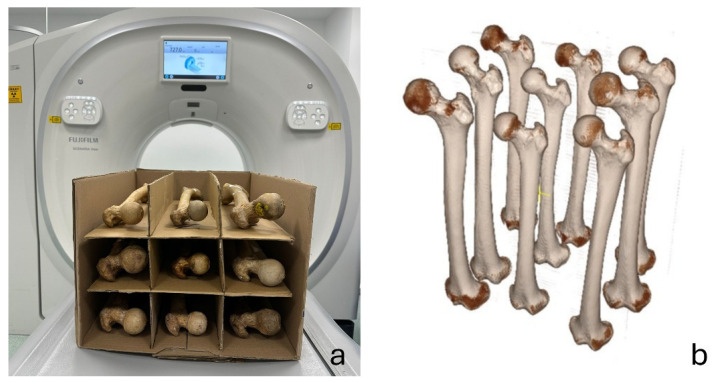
CT scanning setup. (a) Custom-made cardboard box with nine separate compartments used to position the dry femora for simultaneous CT acquisition in the multidetector CT scanner. (b) Three-dimensional volume-rendered reconstruction of the scanned femora generated from the CT data.
